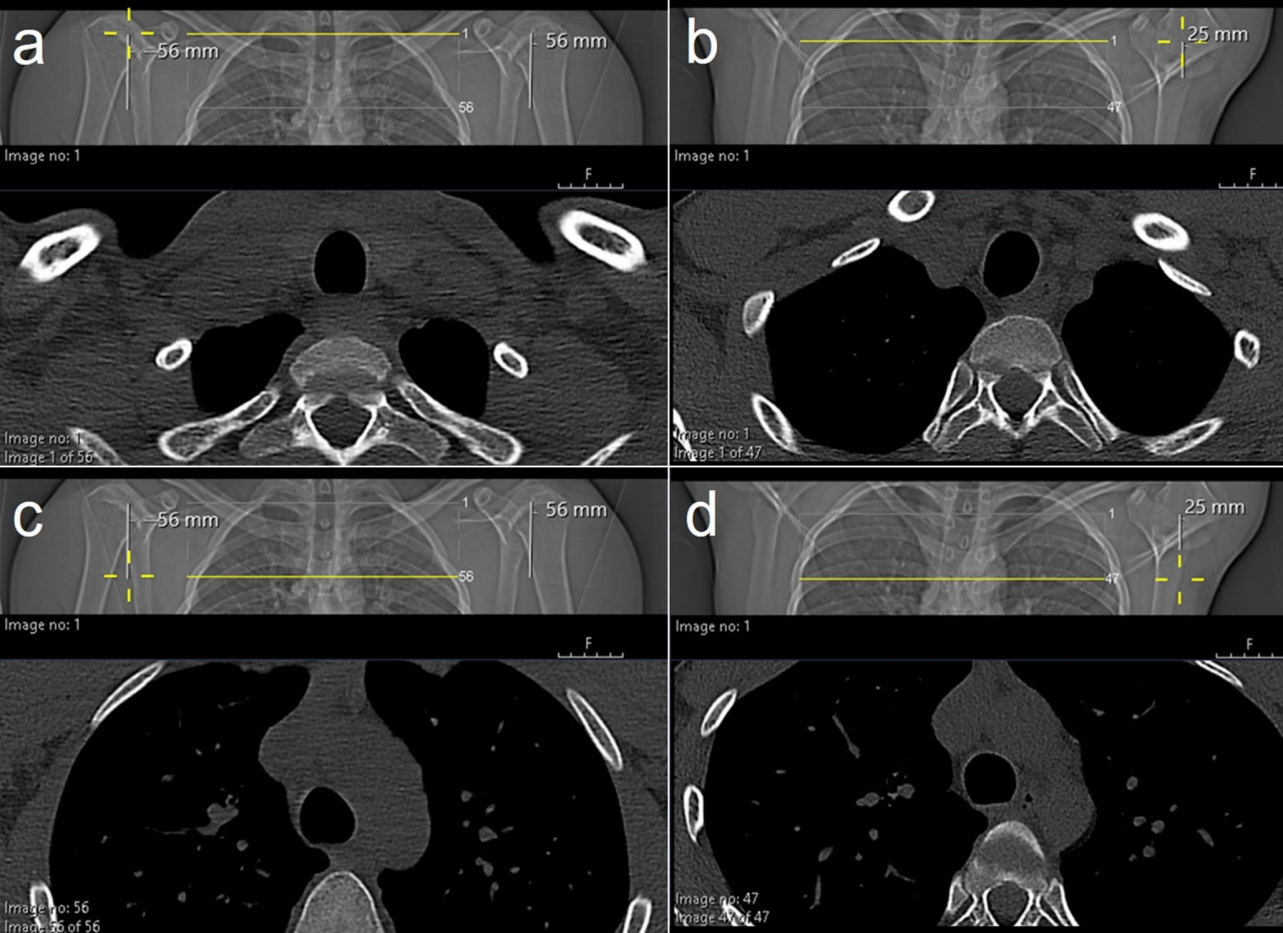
Measurement of length of humeral head/shaft within the beam path. Examples with arms-down (a)/(c) and with arms-up (b)/(d). The humeral hear/shaft length within the beam path is measured on scout views. The cross-hair tool is used to accurately determine the position on the scout view. In arms-down CTs, the measurement extends from the level of the first axial CT image (a) to the last axial image (c). In arms-up CTs, the measurement extends from the level of the first axial CT image (b) to the contour of the humeral head (d). In arms-down CTs, the length of humeral head/shaft within the beam path corresponds to the scan length. In arms-up CTs, the length of humeral head/shaft inclusion in the beam path may vary between the left and right arm. In the above presented examples, the sum humeral head/shaft included in the beam path was 112 mm for the arms-down (56 mm + 56 mm), and 25 mm for the arms-up CT (0 mm + 25 mm)
